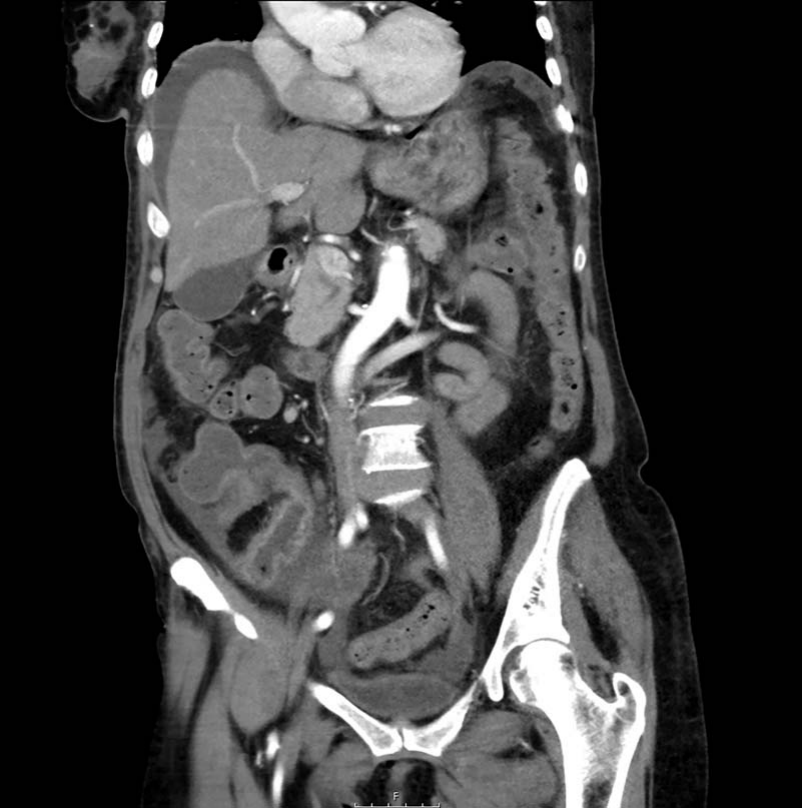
CT scan: sudden change of caliber at the terminal ileum together with signs of intestinal suffering.
